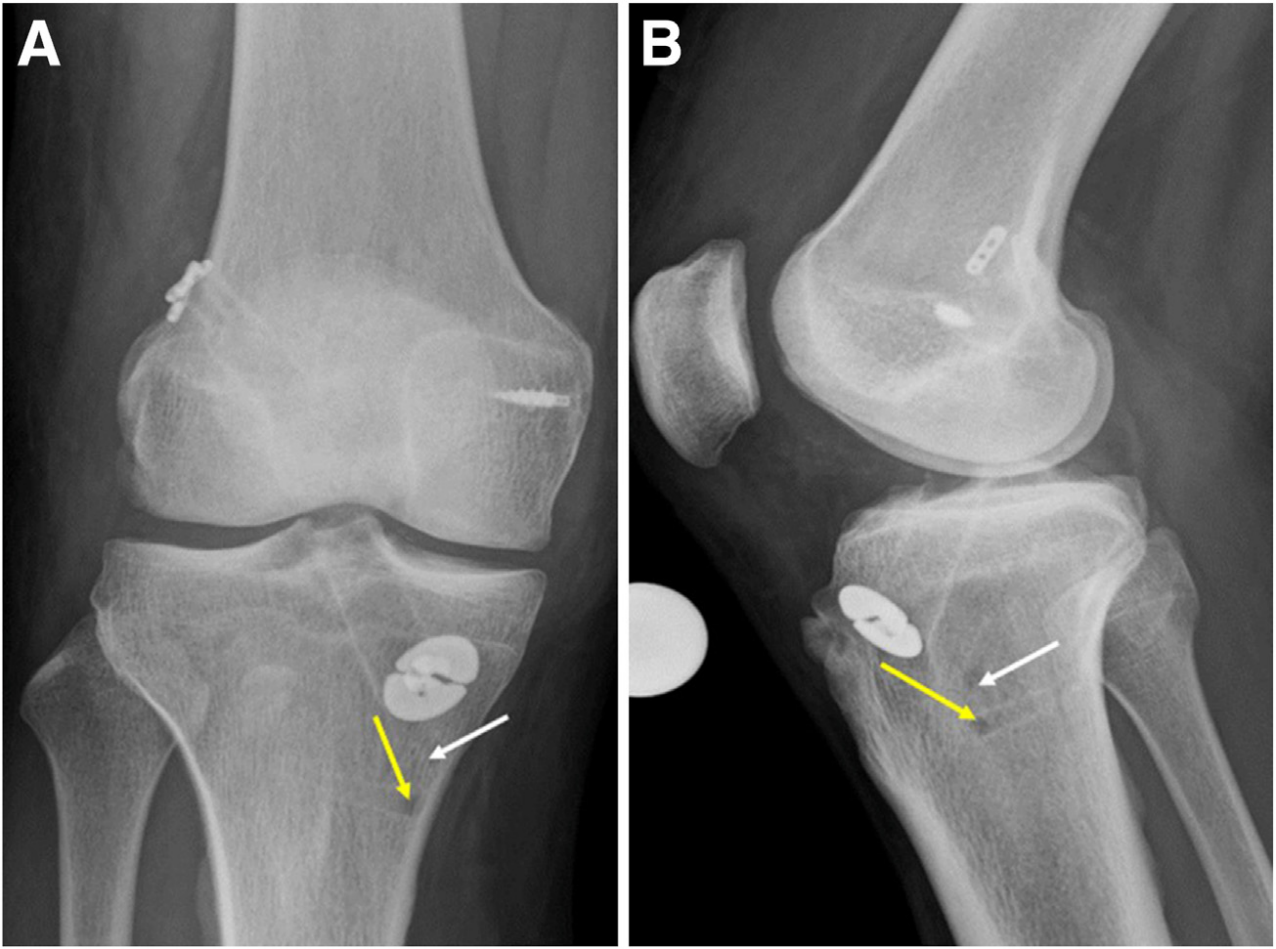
Postoperative radiographs of right knee of patient with new terrible triad with revision ACL reconstruction. (A) Anteroposterior view. A metallic anchor was used for MCL repair during previous surgery. (B) Lateral view. The lateral meniscus root tunnel depicted by white arrow. The root sutures are secured with a suture anchor distally (yellow arrow). The reconstructed ACL graft is fixed with a button. (ACL, anterior cruciate ligament; MCL, medial collateral ligament.)
